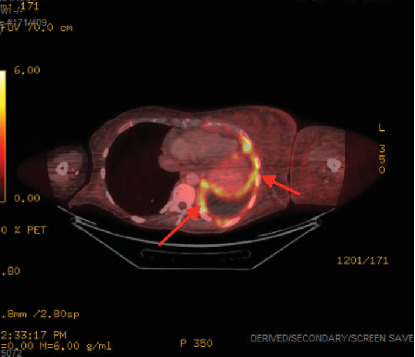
PET-CT (axial). Persistent left lung pleural thickening with increased activity.
